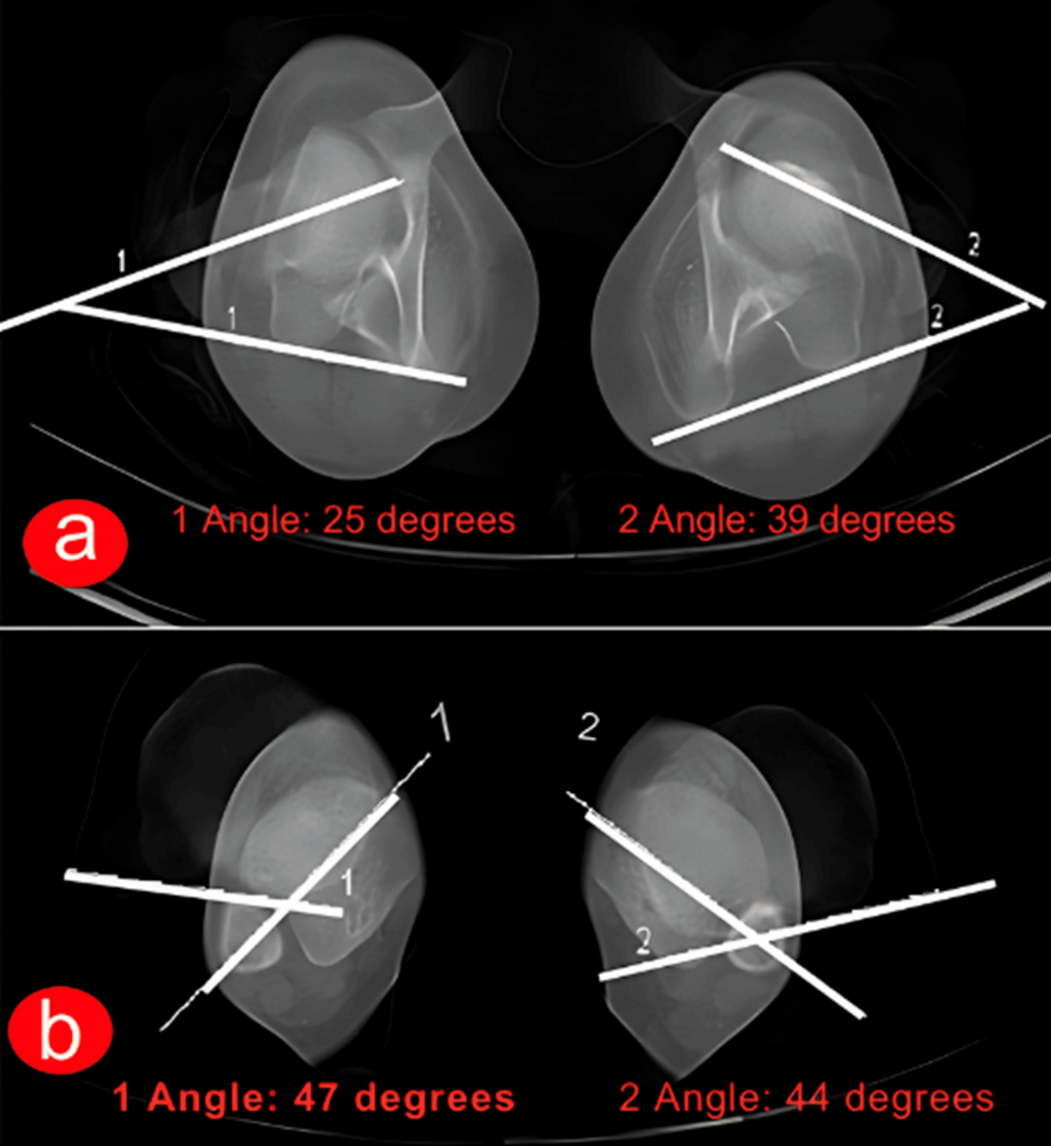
A CT scan of the lower limb’s rotation profile. A CT scan is employed to validate both femoral (as shown in Figure 3a) and tibial torsion (as depicted in Figure 3b). Figure 3a indicates a greater degree of femoral torsion on the left side (measuring 39°) compared to 25° on the right side. Conversely, the tibial torsion of both tibias appears to be nearly symmetrical. Figures are provided by the authors.
